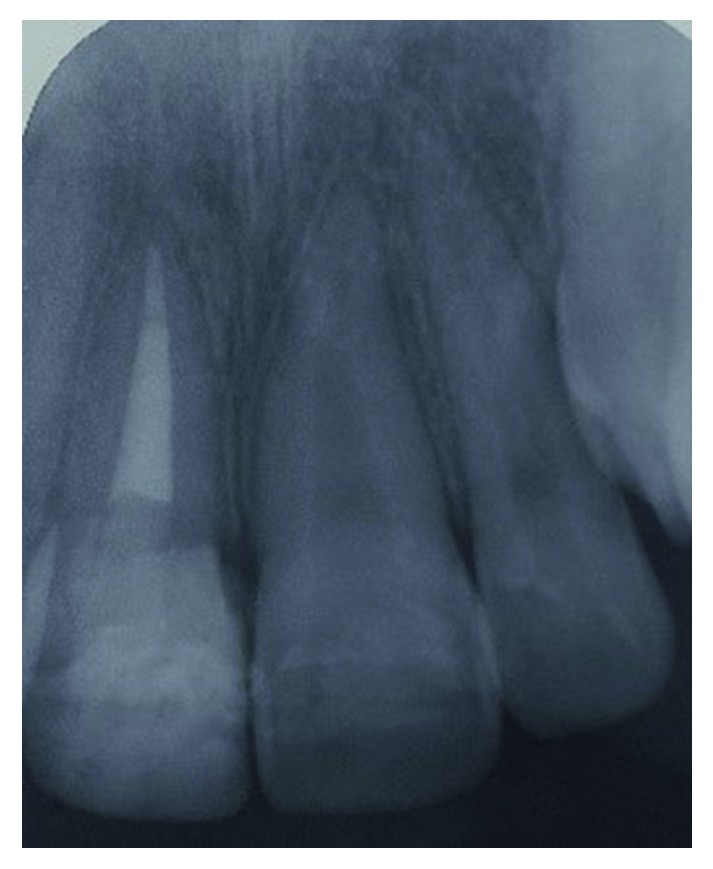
Postoperative periapical radiograph of the fractured tooth 11 at 12 months after extrusion.
